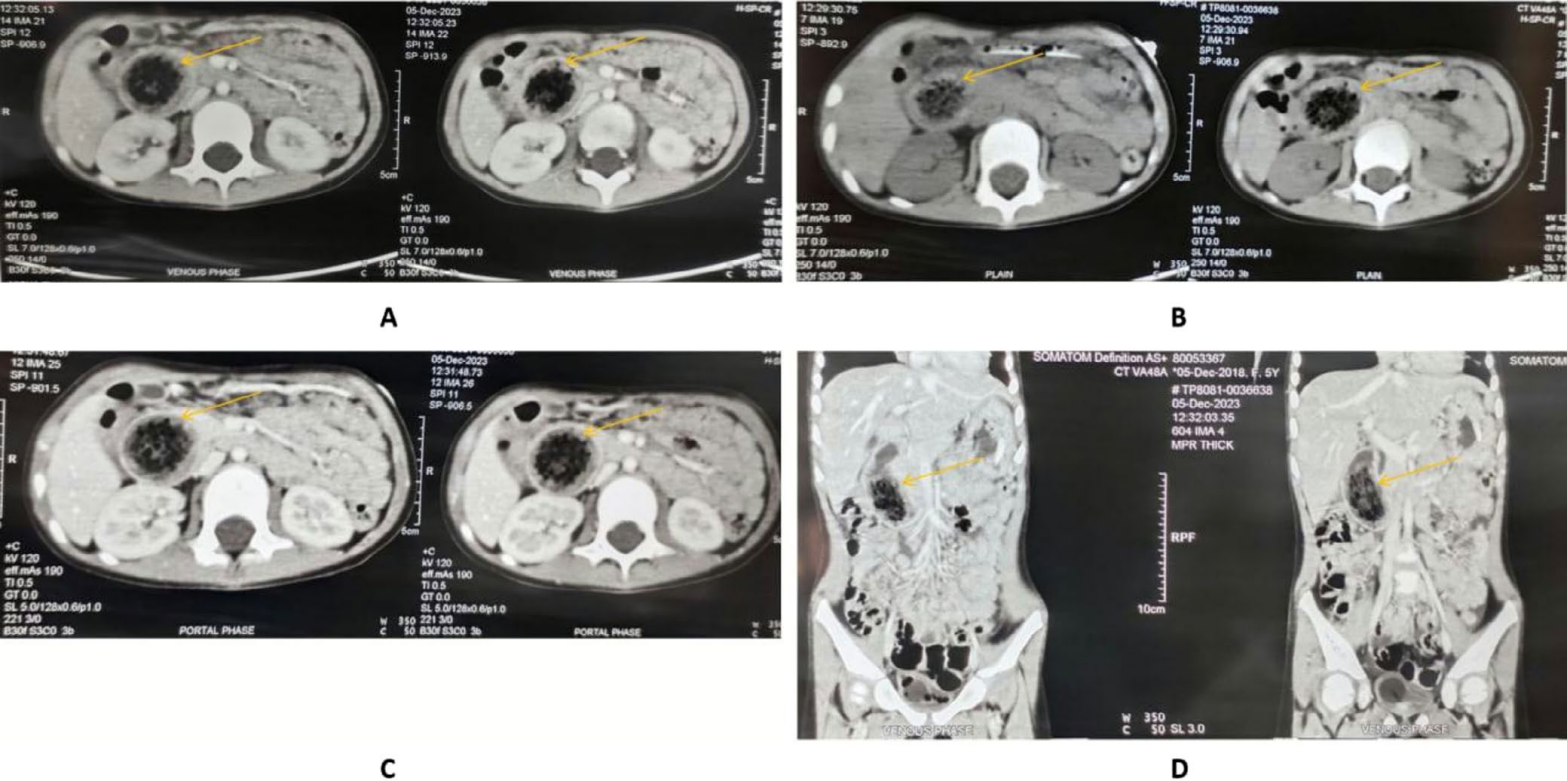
Computed tomography of abdomen showing phytobezoar (arrow shows phytobezoar). (A) Contrast enhanced axial section. (B) Plain axial section. (C) Contrast enhanced axial section. (D) Contrast enhanced coronal section.
